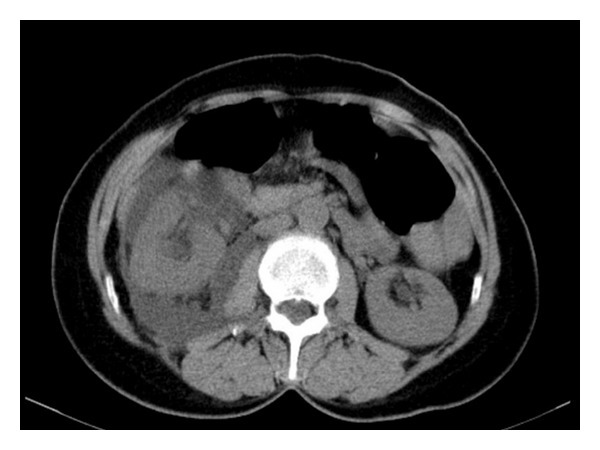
Free fluid around the kidney, especially near the pelvis renalis, is seen at CT scan without contrast-enhanced.
